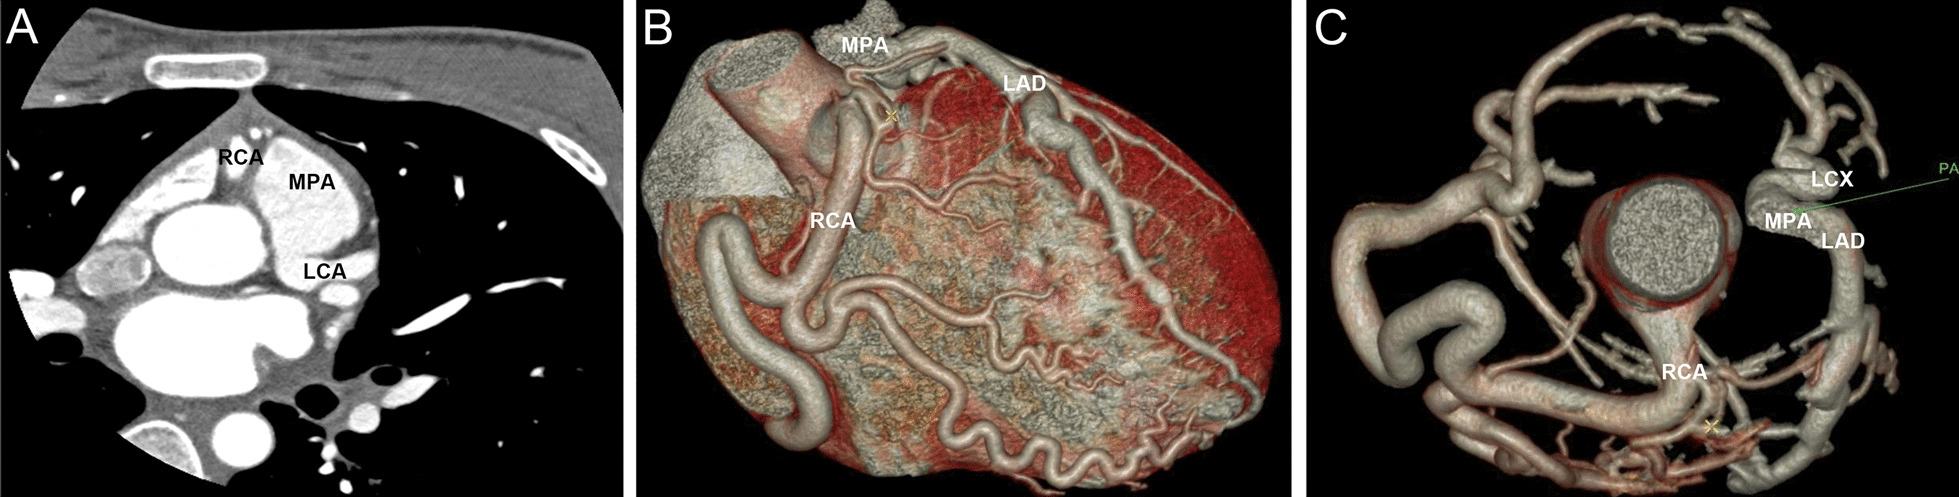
Coronary computed tomography angiography showing the origin and course of the left coronary artery
